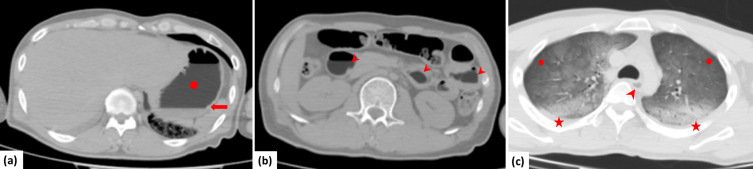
PMCT of a male who died due to benzine poisoning [(a, b) window width/window level = 520/–150, (c) window width/window level = 1500/–500]. (a) PMCT showing two separate fluid layers in the stomach (arrow). The upper layer showed a low density (circle). (b) A similar low-density fluid was observed in the duodenum and jejunum (arrowheads). (c) Low-density fluid was observed in the esophagus (arrow). Bilateral chemical pneumonia (circles) and postmortem hypostasis (stars) were also observed. The CT number of the low-density fluid was approximately −230 Hounsfield units. PMCT, postmortem computed tomography.
